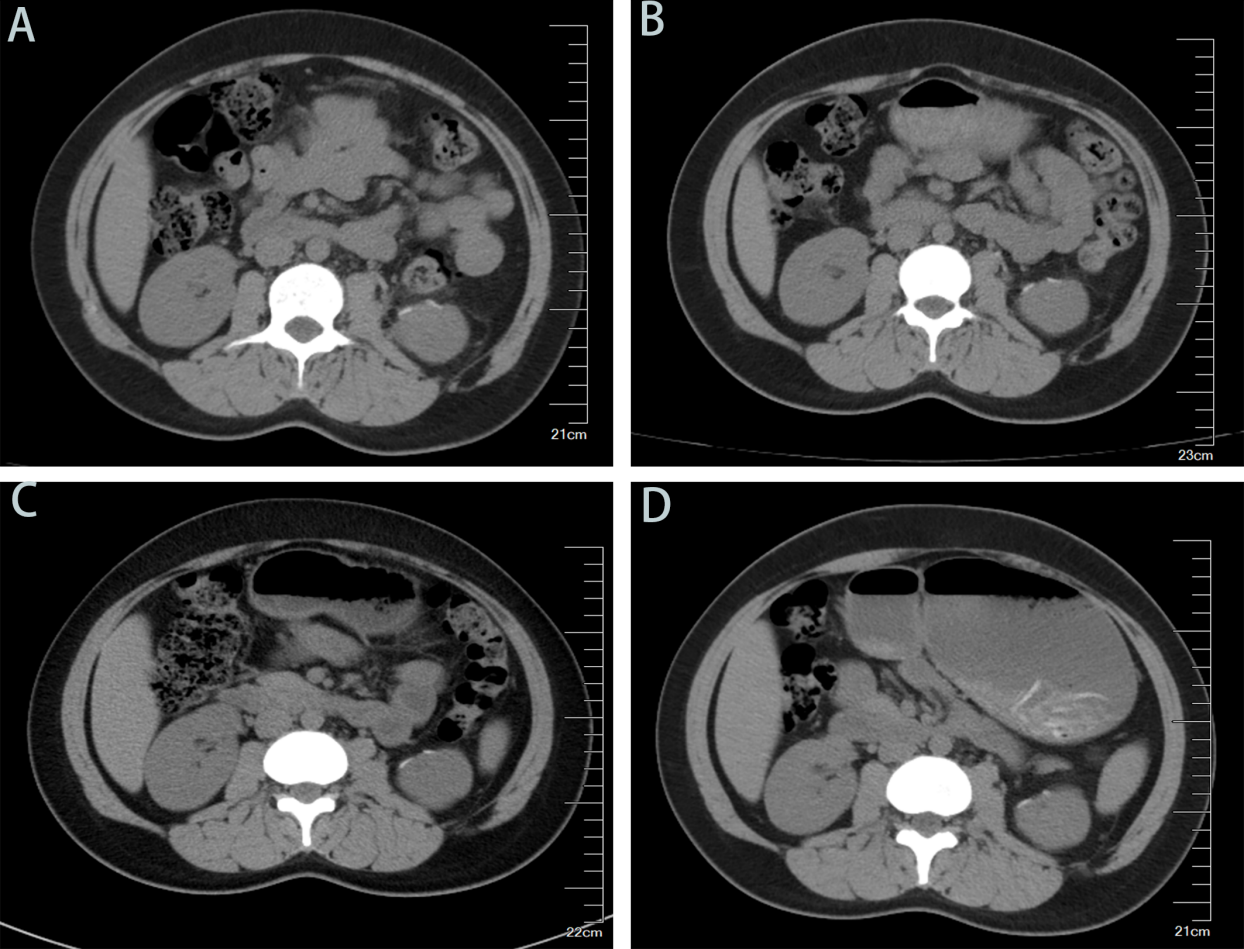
Follow-up Computed Tomography (Non-contrast CT of the Whole Abdomen) Findings. (A) 1-month postoperative non-contrast abdominal CT scan: Showing no evidence of tumor recurrence or metastasis. (B) 4-month postoperative non-contrast abdominal CT scan: Showing no evidence of tumor recurrence or metastasis. (C)7-month postoperative non-contrast abdominal CT scan: Showing no evidence of tumor recurrence or metastasis. (D) 10-month postoperative non-contrast abdominal CT scan: Showing no evidence of tumor recurrence or metastasis.
